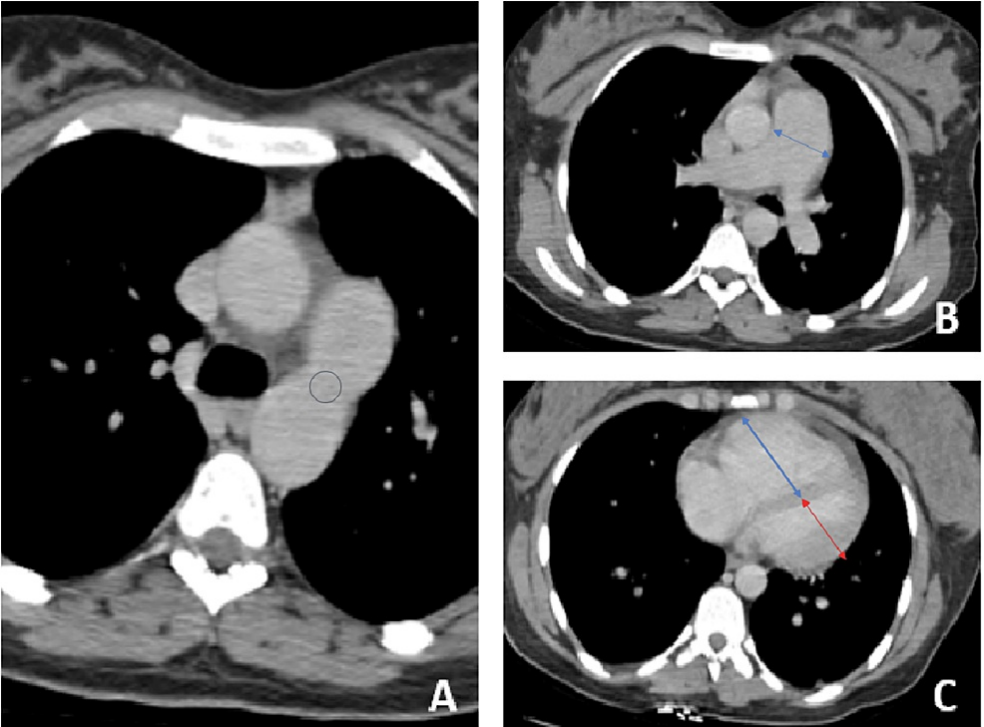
CT pulmonary angiography showed a persistent ductus arteriosus (A, circle), an enlarged pulmonary artery (B, blue arrow), and dilated right ventricle (blue arrow) compressing the left ventricle (red arrow) (C)
